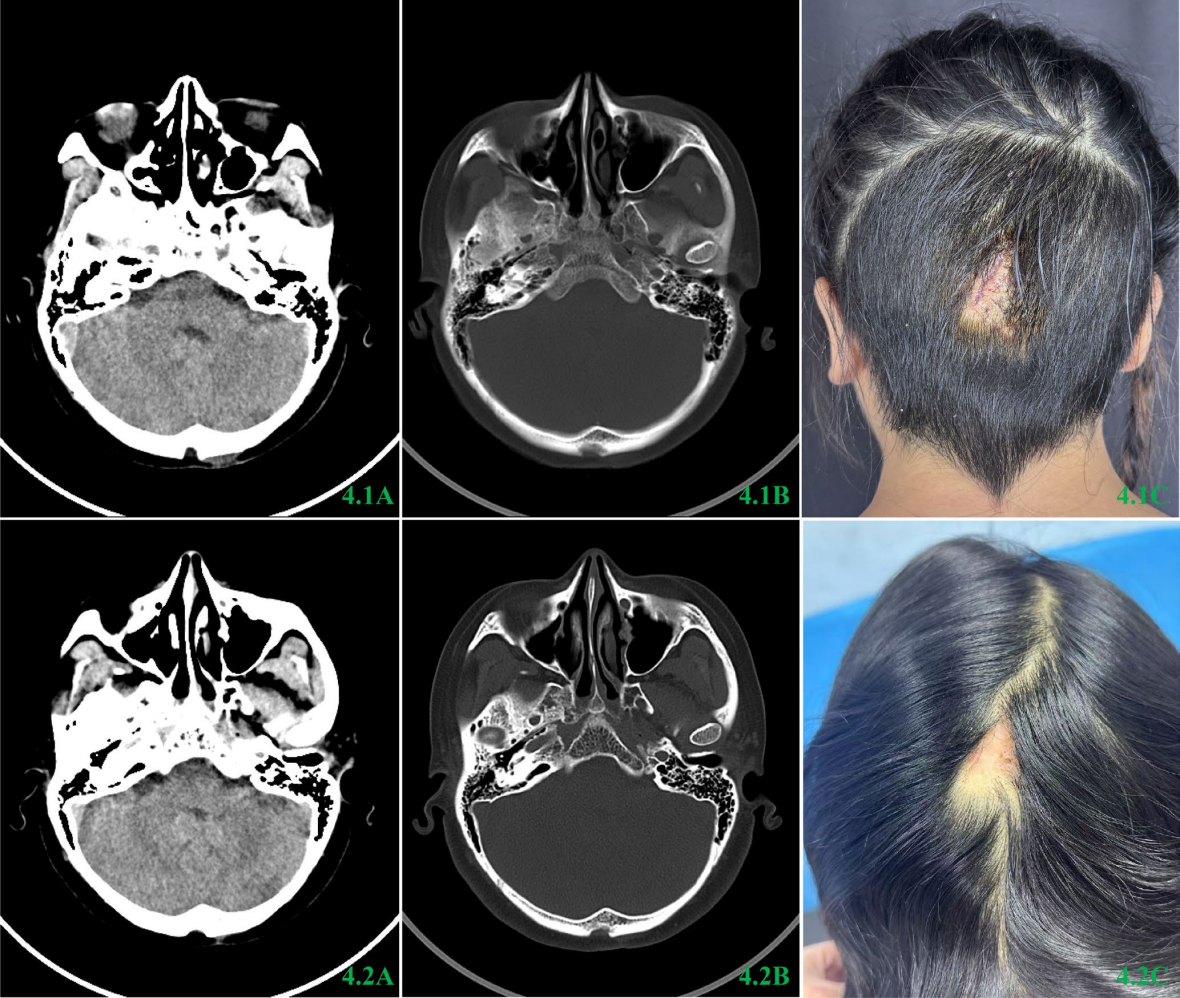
4.1A–C and 4.2A–C refer to 14 days and 10 months after the secondary wound closure, respectively. In 4.1A and 4.2A, CT showed that subcutaneous occipital and occipital lesions had been cleared; no definite abnormality was found in the brain parenchyma. In 4.1B and 4.2B, a short strip defect was observed near the outer plate of the occipital bone. In 4.1C and 4.2C, the incision was anastomosed and healed well.
